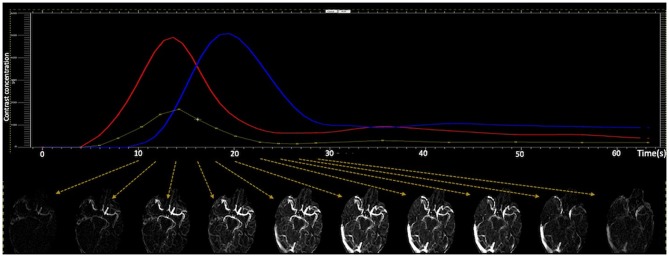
Generating dynamic CTA images. Ten continues CTA images were manually selected from CTP source images, which covers early artery phase, peak arterial phase, late arterial/peak venous phase, and late venous/washout phase.
